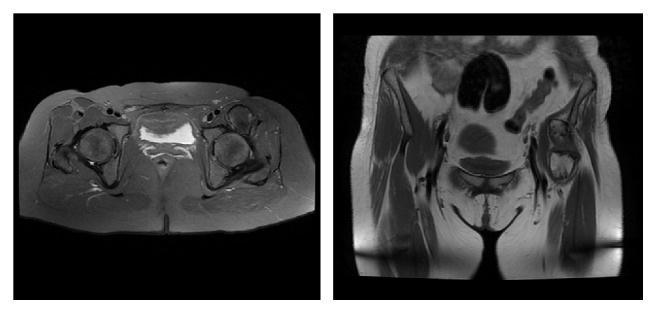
Preoperative radiographic evaluation MRI.
